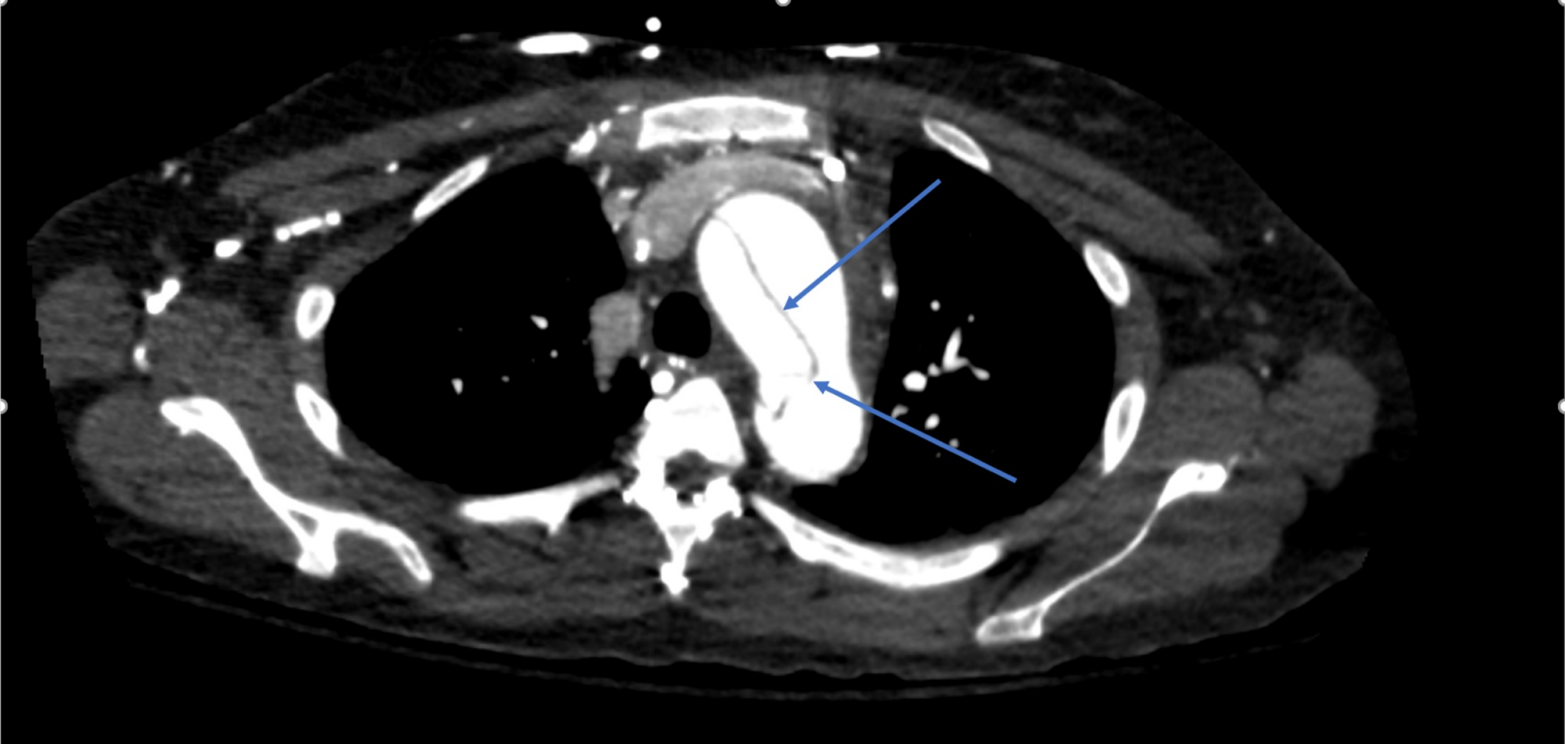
CT scan showing aortic dissection
